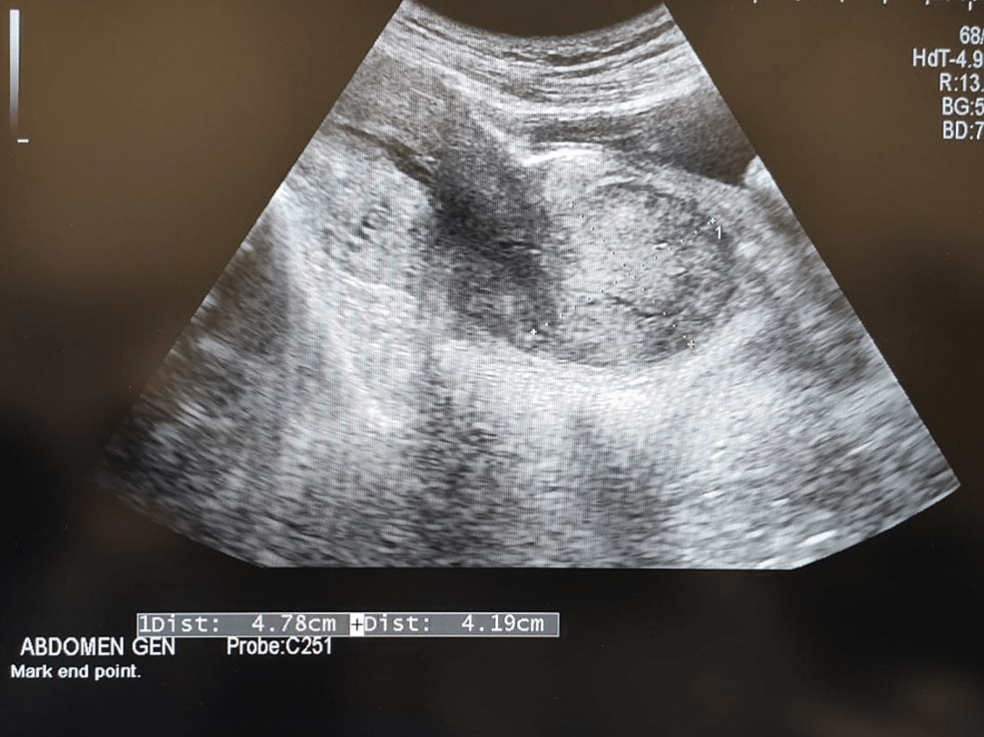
USG dimensions of fibroid USG: ultrasonography
